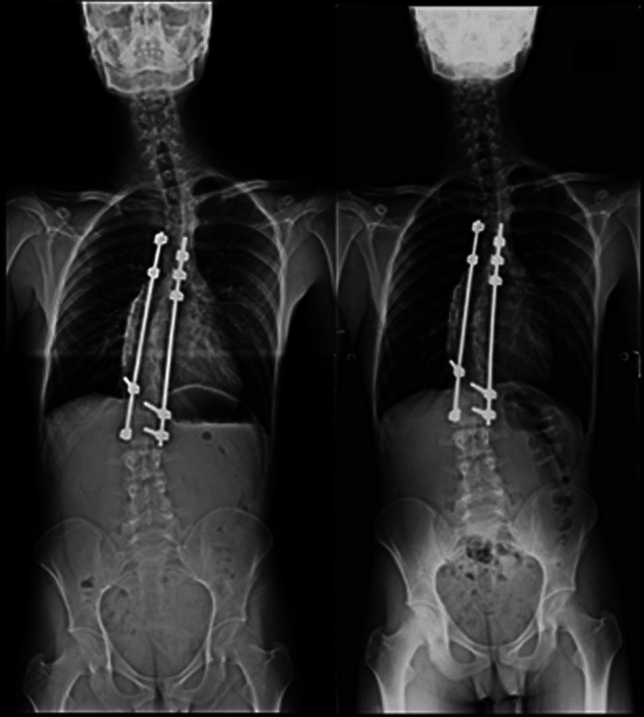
An example of no-correction of PSI. (Left) Immediate postoperative standing whole-spine frontal radiograph of an AIS patient revealed PSI. (Right) Whole-spine frontal radiograph at 1 year after operation in the same patient revealed no correction of PSI
